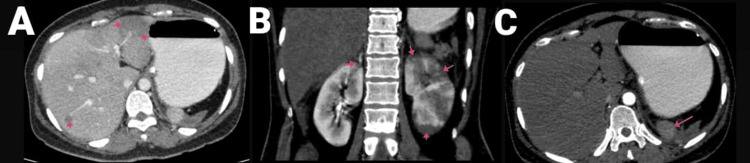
CECT of the abdomen and pelvis with angiography of the patient, with arrows showing (A) hepatic infarcts, (B) renal infarcts, and (C) shrunken spleen with splenic infarct. CECT, contrast-enhanced computed tomography
